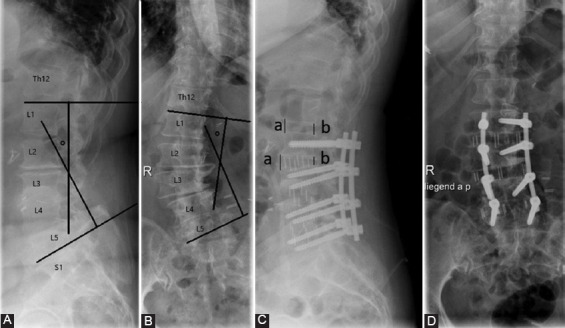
A. Preoperative lateral X-ray of the lumbar spine. Technique of assessment of L1-S1 angle (lumbar lordosis; angle between the upper endplate of L1 and upper endplate of S1 vertebra). B. Preoperative coronal X-ray of the lumbar spine. Technique of assessment of L1-L5 angle in the coronal plane (angle between the upper endplate of L1 and lower endplate of L5 in coronal plane). C. Postoperative lateral X-ray following three-level XLIF in L2/3, L3/4, L4/5 with supplemental fixation L2-5. Depiction of disc height assessment as an average of anterior and posterior disc height. D. Postoperative coronal X-ray shows increased disc height.
